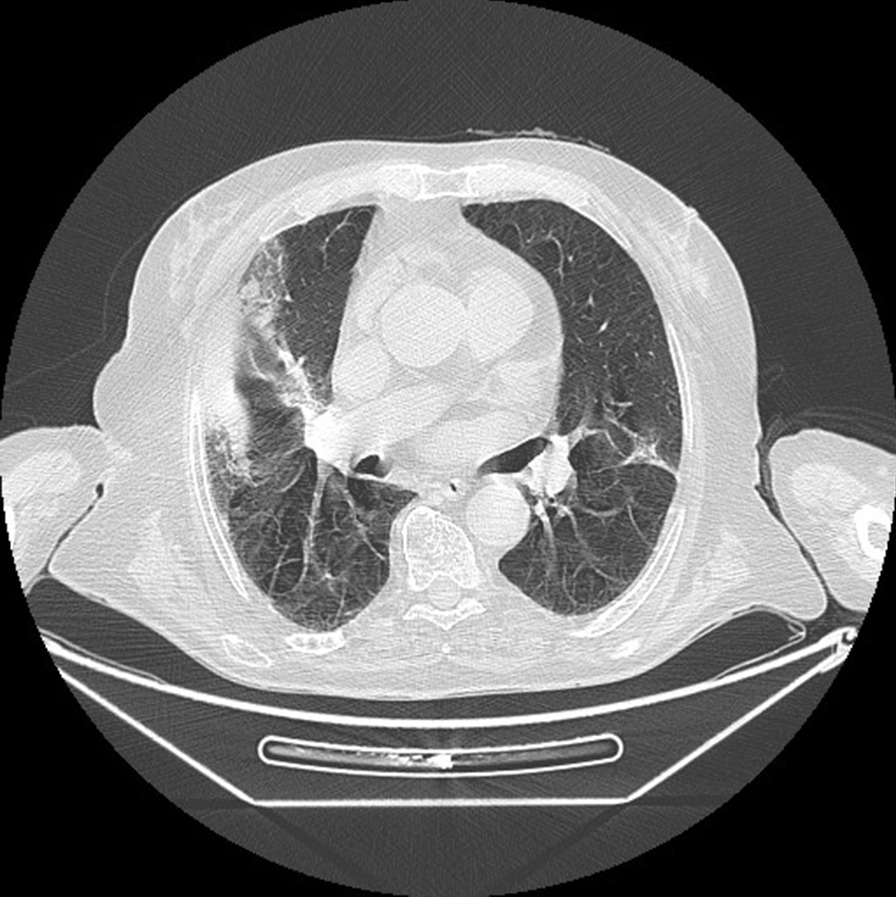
Chest computed tomography image showing ground-glass opacity, crazy paving, and consolidation
